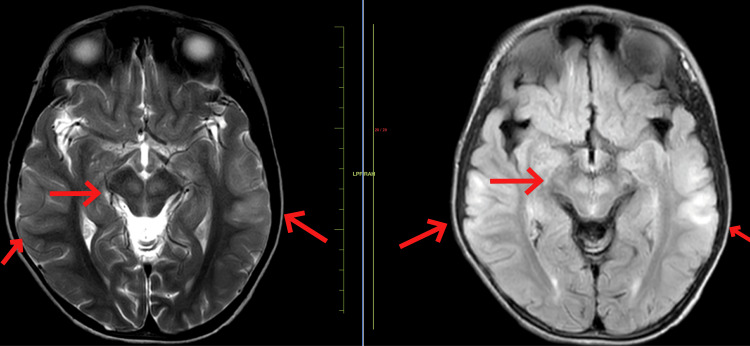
MRI showing T2/FLAIR hyperintensities noted in bilateral red nucleus, superior and inferior colliculus and periaqueductal region. MRI: Magnetic resonance imaging; FLAIR: fluid-attenuated inversion recovery
